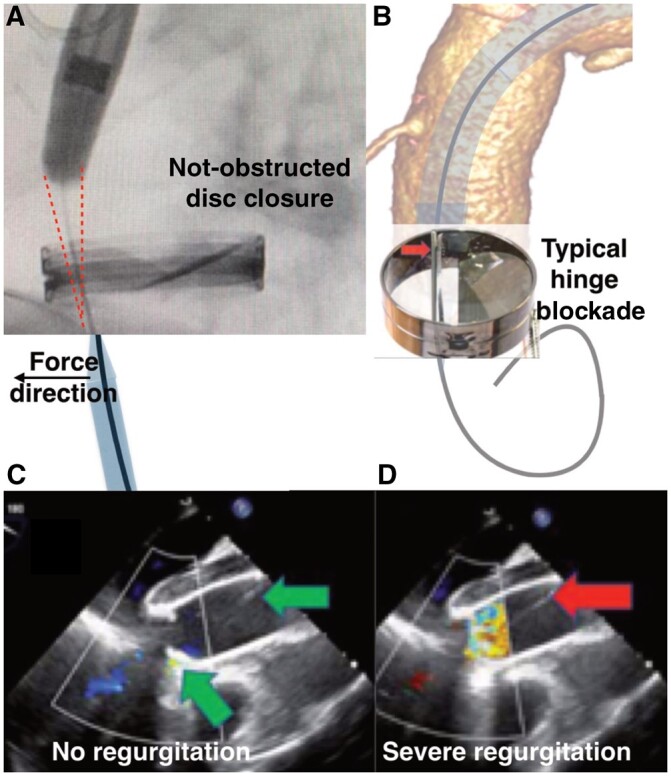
(A) Red sketch on the fluoroscopic image shows space obtained after cutting 15 mm from the tip of the stent graft. The wire was guided by the 6 Fr sheath from the apex to keep it away from the disc hinge. (B) 3-Dimensional reconstruction of the tomographic image. Mechanism of the disc blockade if the valve is simply crossed. (C/D) Transoesophageal echocardiography. (C) Effective valve closure; green arrows show correct position of the wire. (D) Severe valve regurgitation after the blockade of the disc. Red arrow shows the position of the wire.
